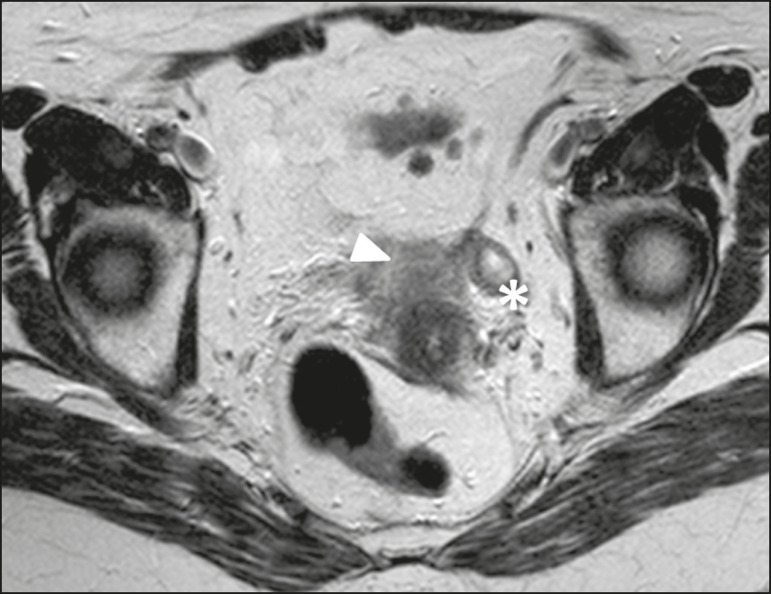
Müllerianosis of the left broad ligament in a 74-year-old woman. Axial T2-weighted imaging showing a tumor with similar characteristics with the uterus (asterisk) suspended from the broad ligament and clearly demarking the uterus (triangle).
